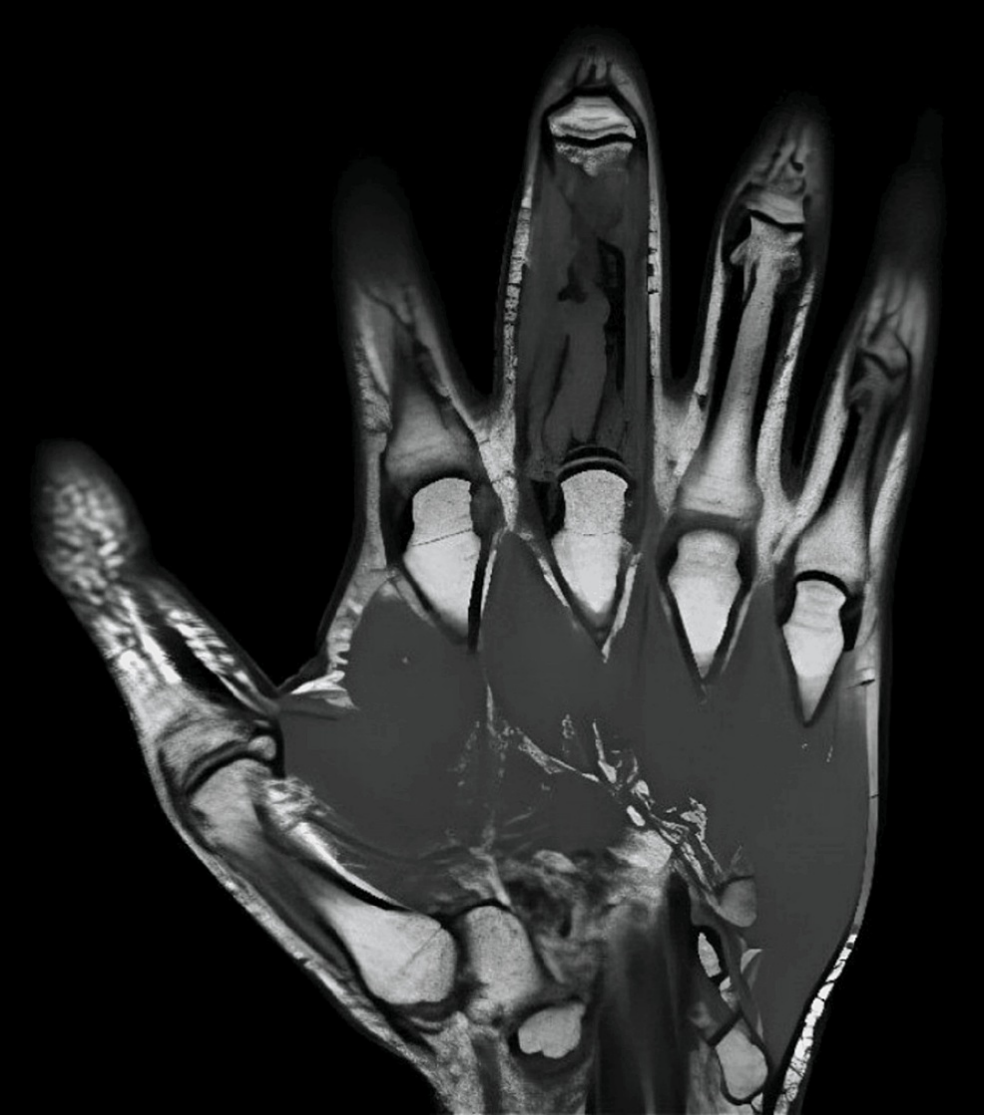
Coronal T1-weighted magnetic resonance imaging of the right hand (2020)
